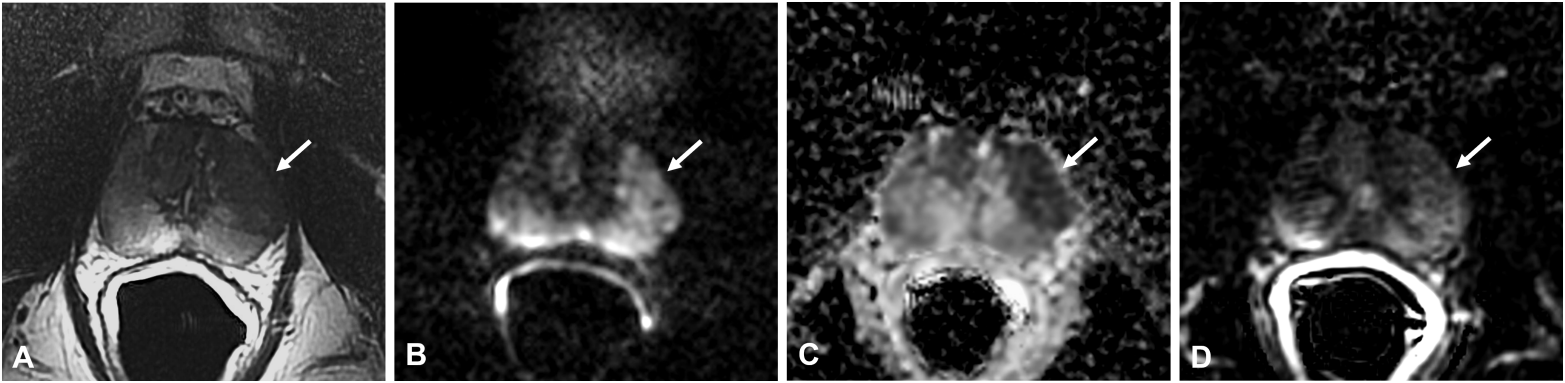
Non-specific granulomatous prostatitis in a 47-year-old patient with a PSA value of 9.81 ng/ml. The axial T2-weighted sequence shows a large hypointense area in the anterior left peripheral zone (PZ) with bulging of the glandular capsule (arrow in A) with diffuse hyperintensity on the DWI image (arrow in B), marked hypointensity on the ADC map (arrow in C), and diffuse mild enhancement on the DCE image (arrow in D).
